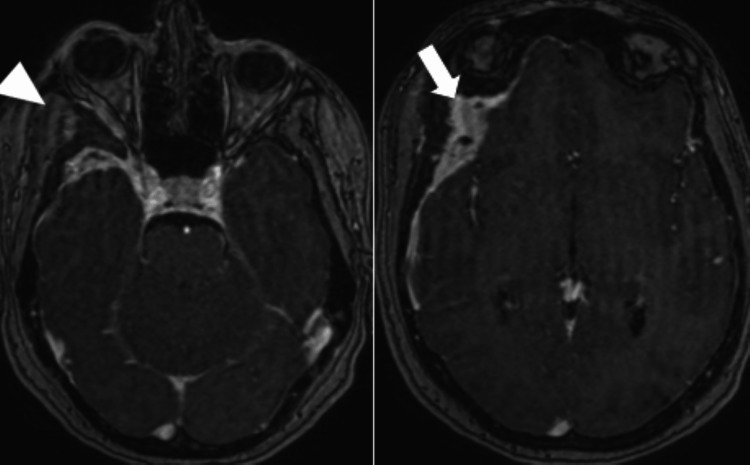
Brain MRI axial sequence Right spheno-fronto-temporo-orbital mass (arrowhead) with thickening and meningeal enhancement opposite (arrow).
